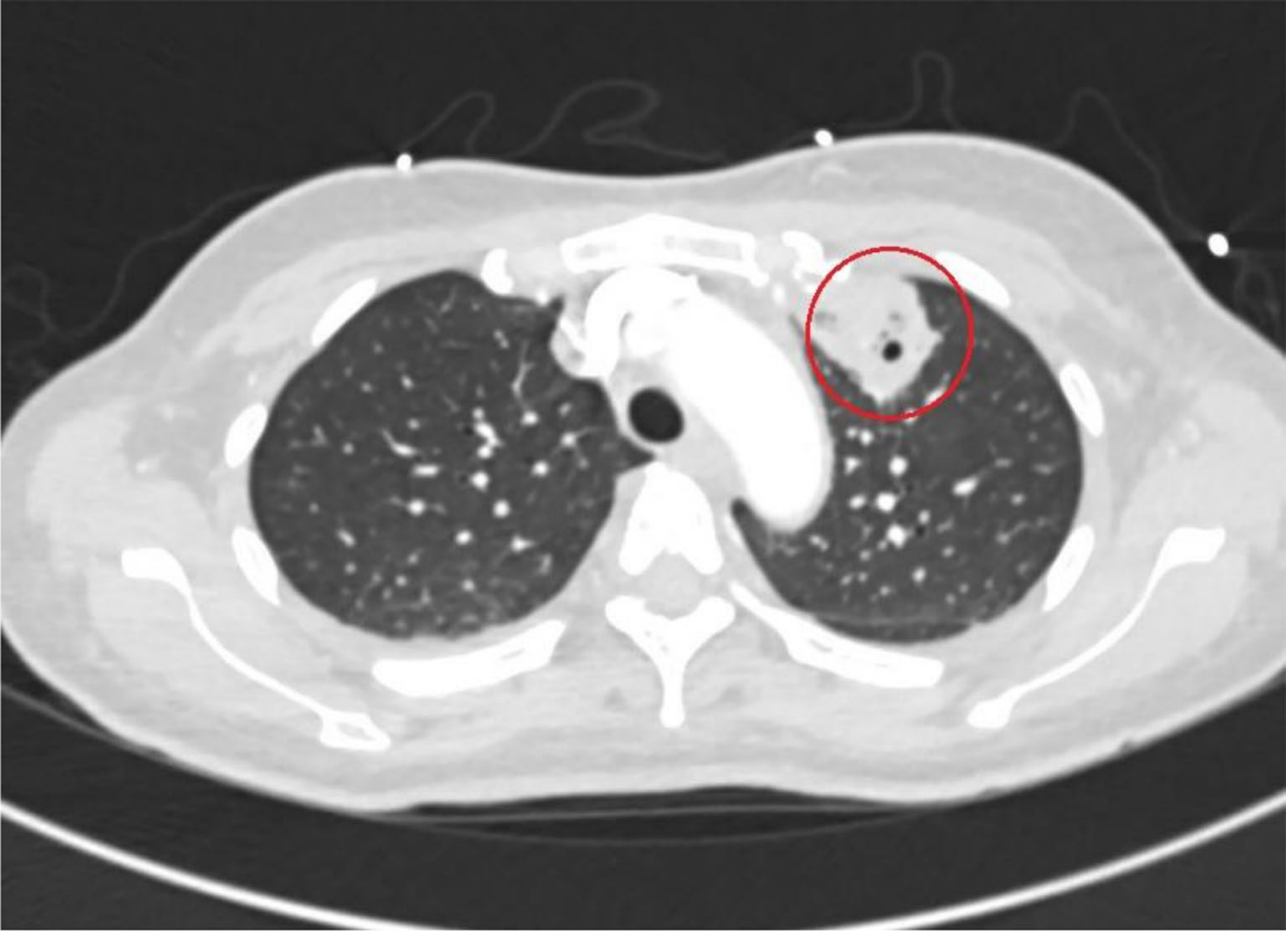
CT scan of the chest at level of aortic arch (day of admission): cavitary lesion in the left upper lobe of the lung
